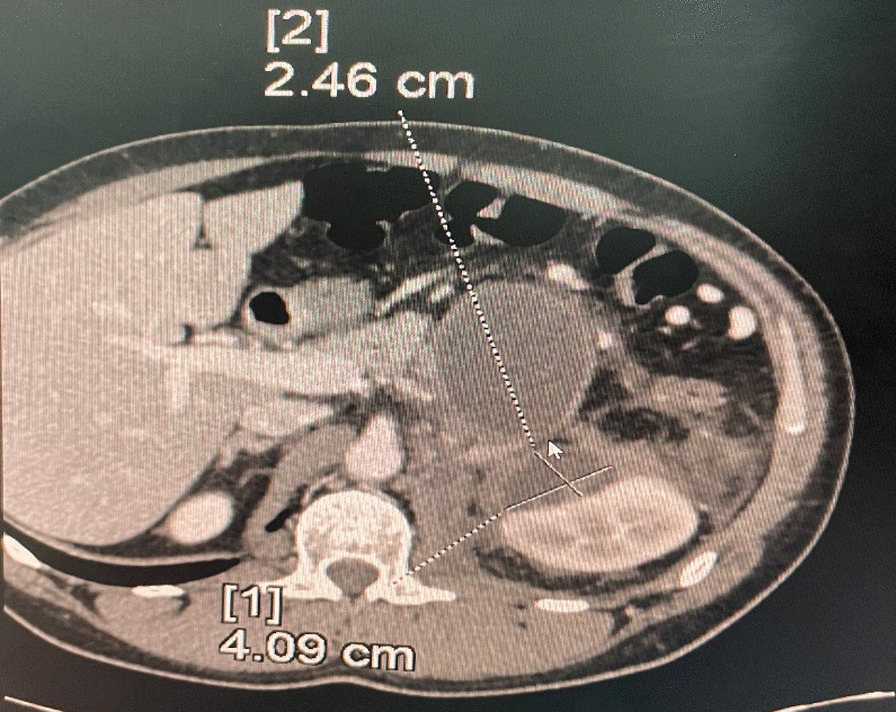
Axial sections of CT scan when the patient first presented to our hospital, showing an important infiltration of the left perirenal and pararenal fat with the presence of a collection of upper pole of the left kidney, which measured 25 × 40 mm and had a liquid density. The collection presented peripheral rim enhancement and the pancreas was within normal size without swelling. The CT also revealed a pancreatic pseudocyst of 68 × 50 mm, which was associated with a discreet dilation of the Wirsung canal
